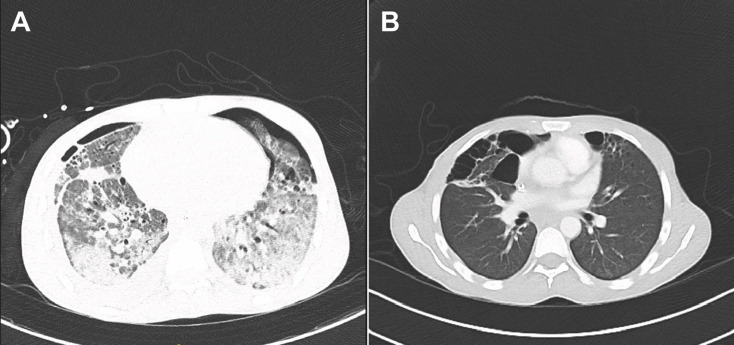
A Chest CT at beginning of spontaneous breathing trials. Progressive, patchy to confluent bilateral consolidations, most pronounced in the lower lobes, with underlying bronchiectasis in the setting of TB-related ARDS. Increasing ground-glass opacities in the upper lobes. New pleural effusions (right > left). On the right, air inclusions and adjacent pleural thickening are present. In addition, a new small ventral pneumothorax is present on the left side. B Follow-up chest CT on the medical ward. New, marked bullous changes with interval improvement of prior consolidations and ground-glass opacities
